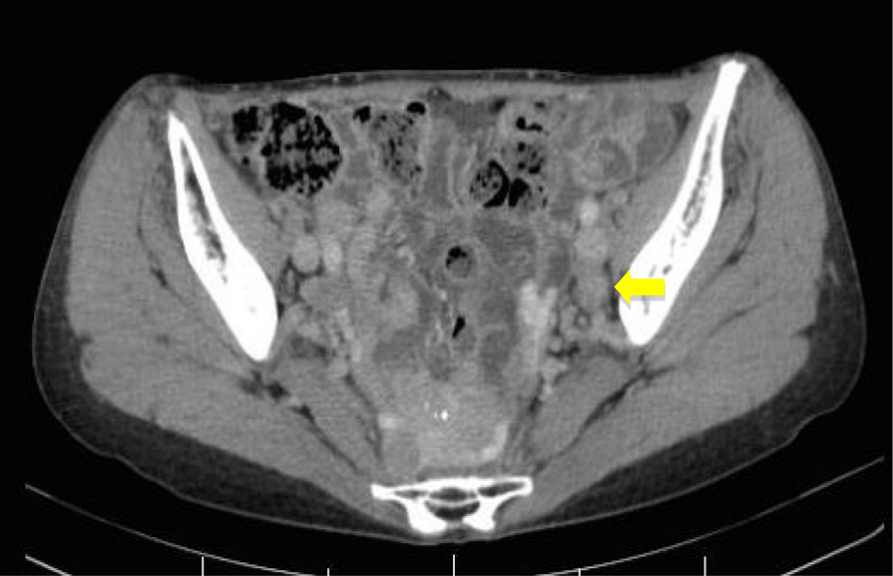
Abdominal CT scan 12 months after surgery showed a lymph node swelling at the left external iliac region (arrow)
